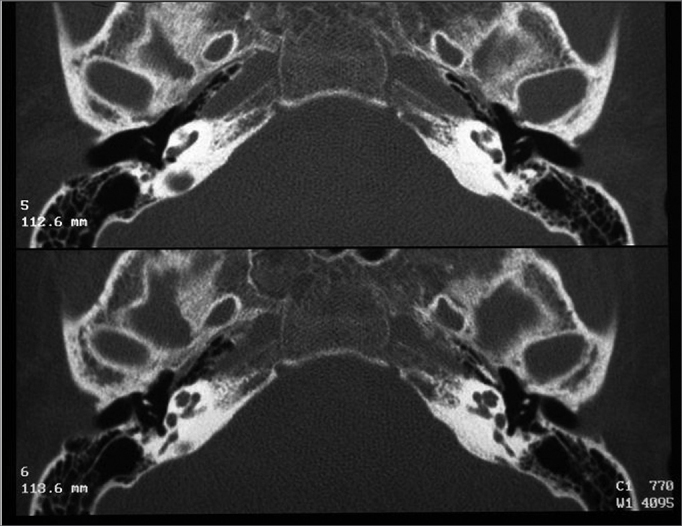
Normal bilateral vestibular aqueduct - Temporal bone CT scan - axial view.
